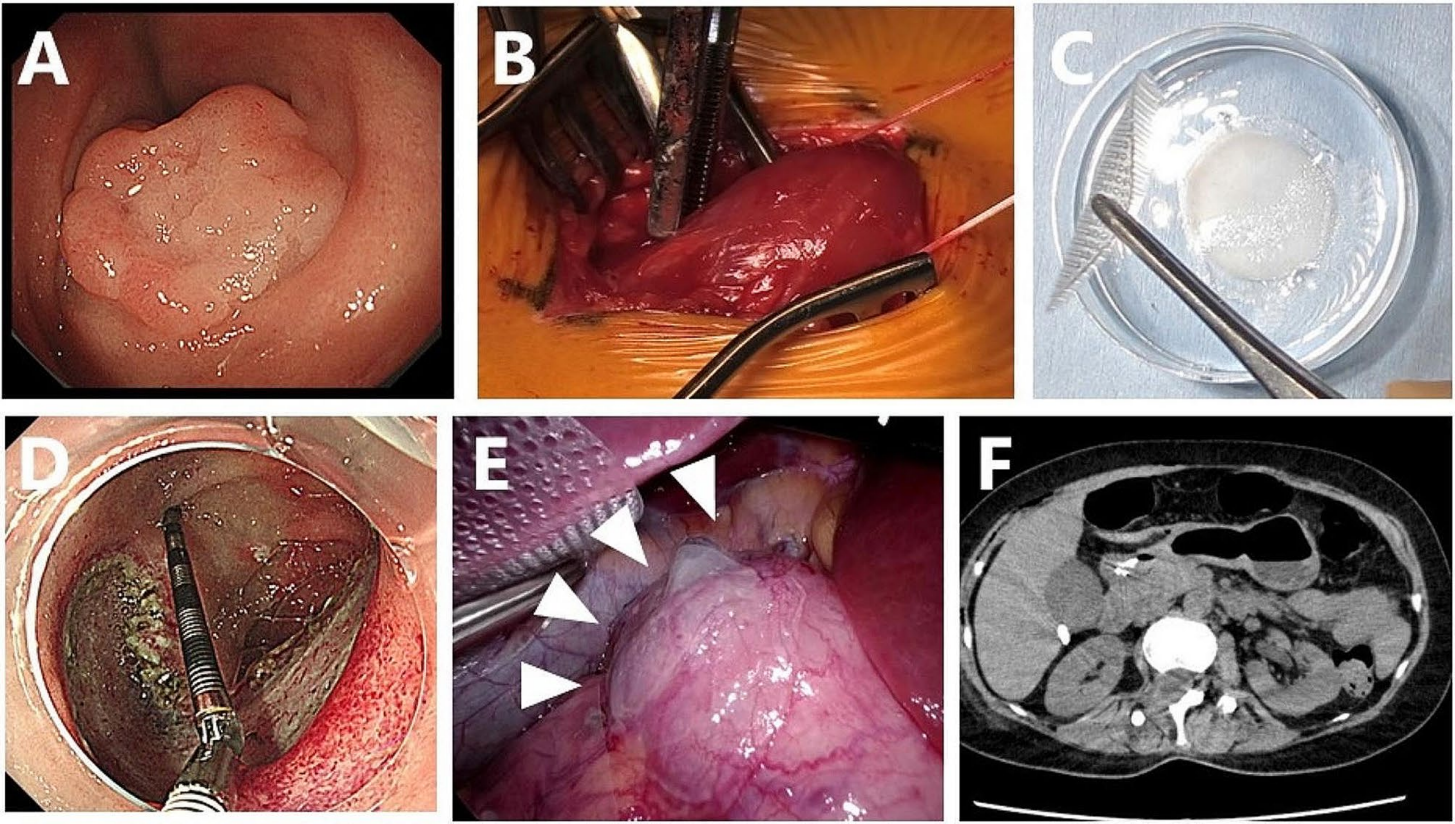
Preparation of the myoblast sheet and laparoscopic transplantation of the sheet after ESD in case 1. A: Superficial duodenal tumor located in the second portion of the duodenum. B: Muscle tissue was surgically excised from the femur of the patient. C: A transplantable myoblast sheet was harvested after seven weeks of culture. D: A mucosal defect around 40 mm in diameter after duodenal ESD. E: Cell sheets were transplanted onto the serosal side of the duodenal wall after ESD. C: Abdominal CT three days after transplantation revealed no obvious signs of perforation
